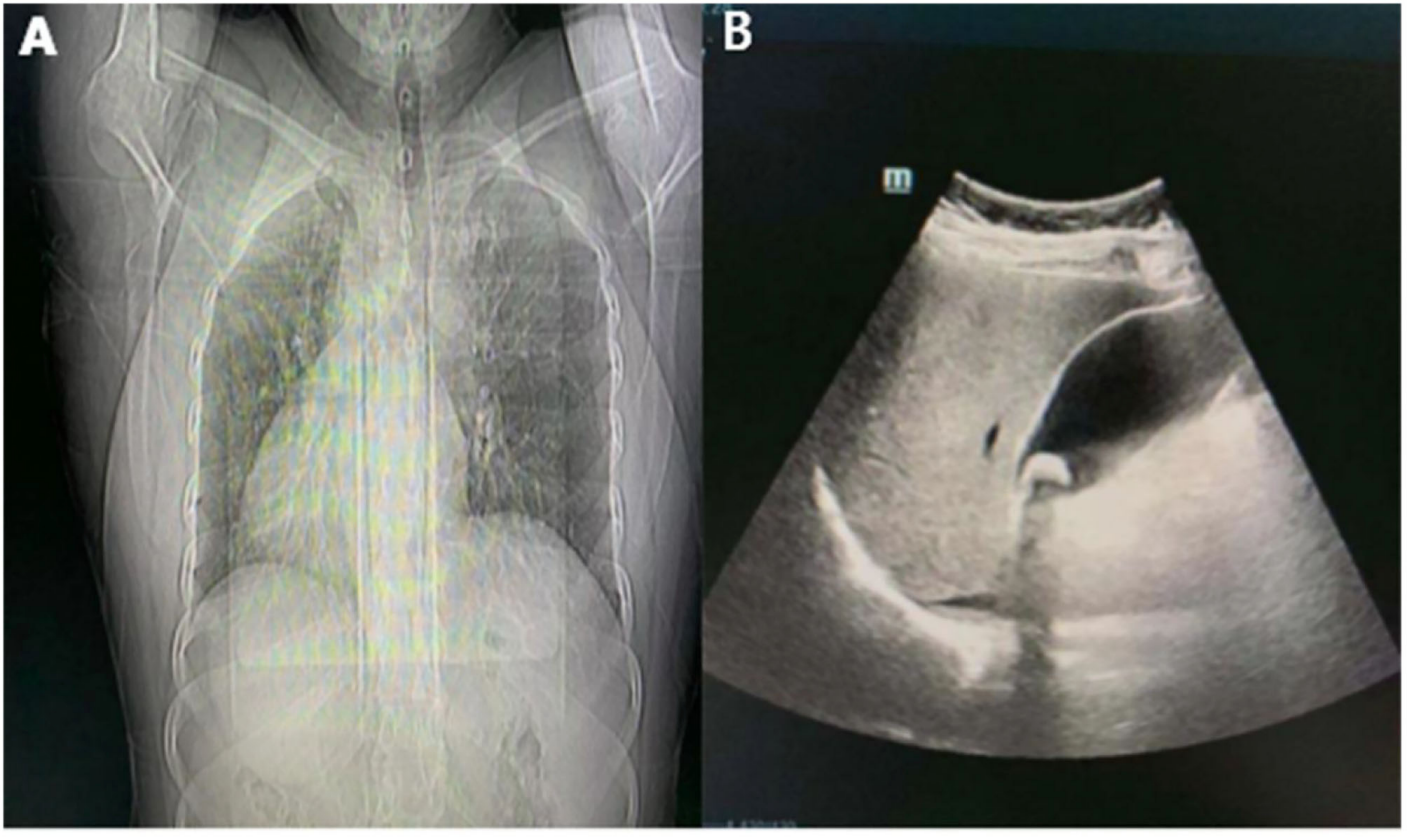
(A) Chest X-ray displays dextrocardia; (B) ultrasound indicated the gallbladder was located in the left upper abdomen with cholecystolithiasis.
